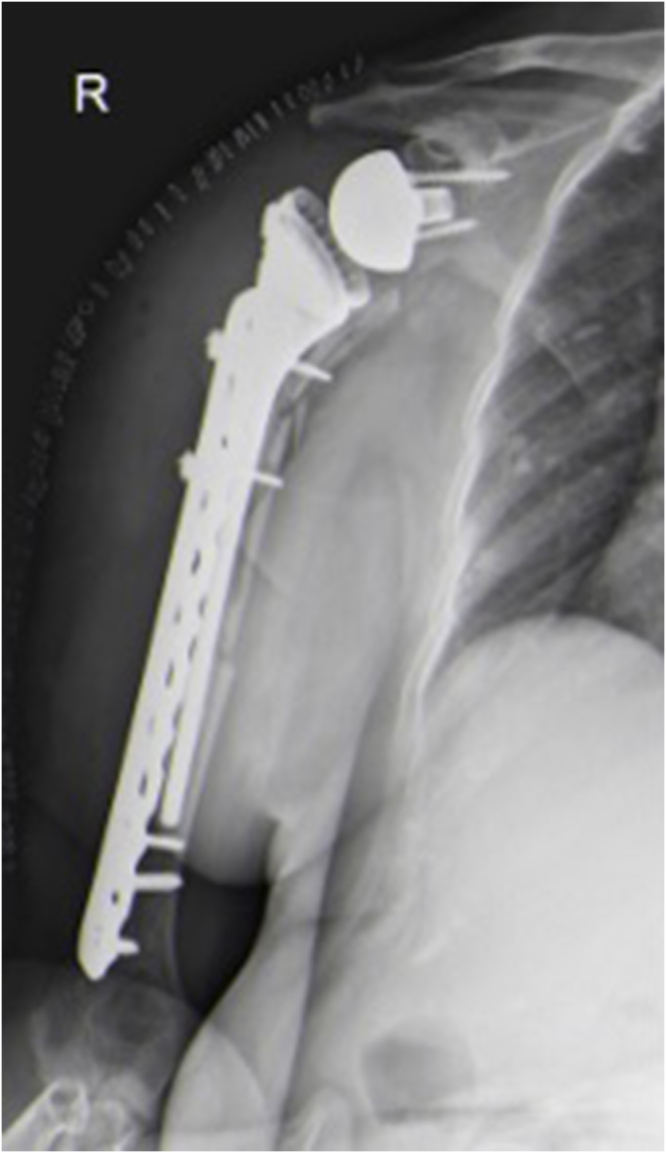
Postoperative X-ray of open reduction internal fixation and long-stem revision of periprosthetic fracture.
